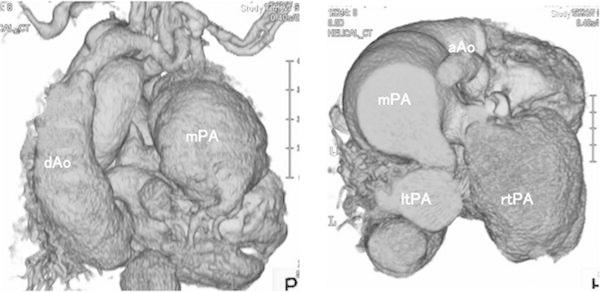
Enhanced computed tomography showed significant dilatation of the bilateral pulmonary arteries and descending aorta. *mPA* main pulmonary artery, *rtPA* right pulmonary artery, *ltPA* left pulmonary artery, *dAo* descending Aorta
